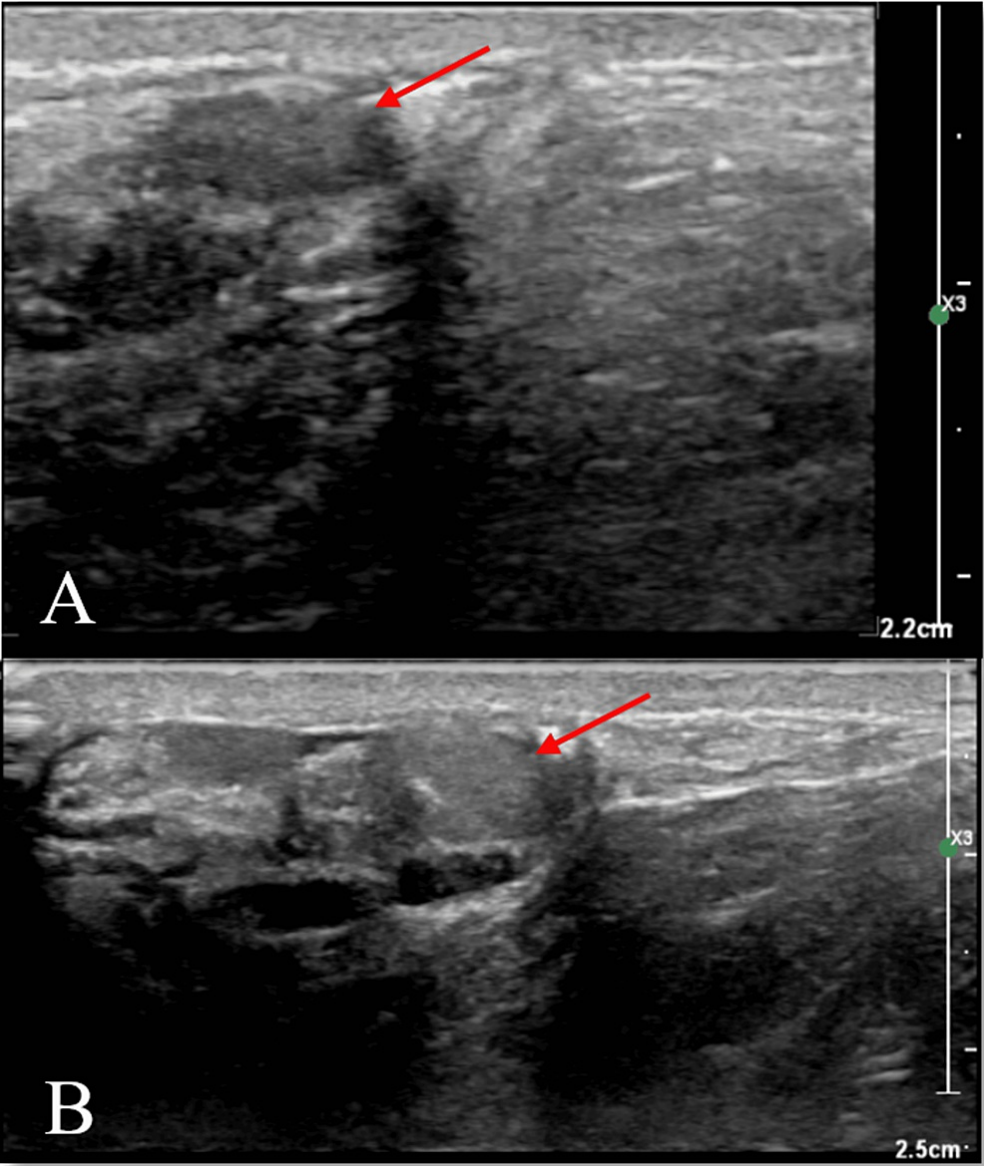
Scrotal ultrasound. (A) The left scrotal ultrasound shows an atrophic left testicle with a measured size of 1.8 cm x 0.7 cm x 1.5 cm and an estimated volume of 1.0 cc. The echogenicity, position, and vascularity of the left testicle and scrotum appear normal.  (B) The right scrotal ultrasound shows an atrophic right testicle with a measured size of 2.0 cm x 0.7 cm x 1.2 cm and an estimated volume of 0.9 cc. The echogenicity, position, and vascularity of the right testicle and scrotum are normal.
